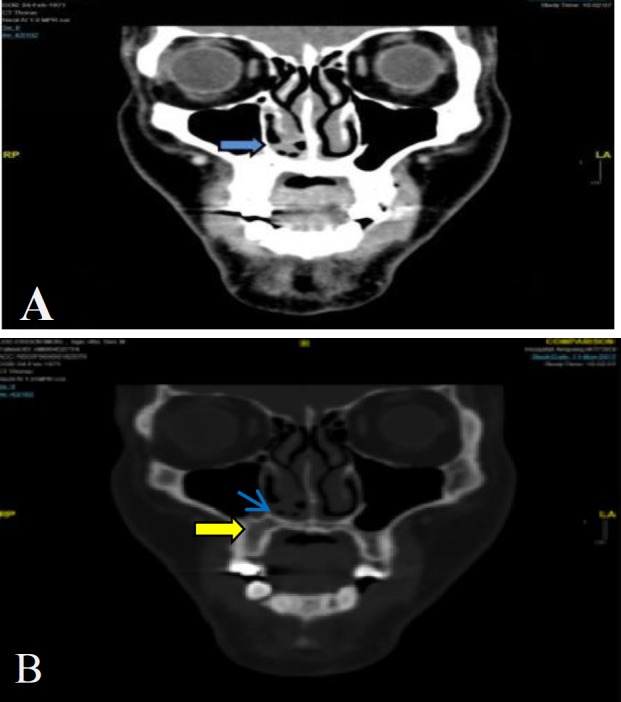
A) Contrast-enhanced computed tomography (CT) neck soft-tissue window coronal view of patient (blue arrow showing a soft tissue mass at the floor of right anterior nasal cavity), B) contrast-enhanced CT neck bone window coronal view of patient) yellow arrow showing the mucosal thickening in right maxillary sinus, blue arrow showing same soft tissue mass at the floor of right anterior nasal cavity, no bony erosion was seen adjacent to the tumor)
